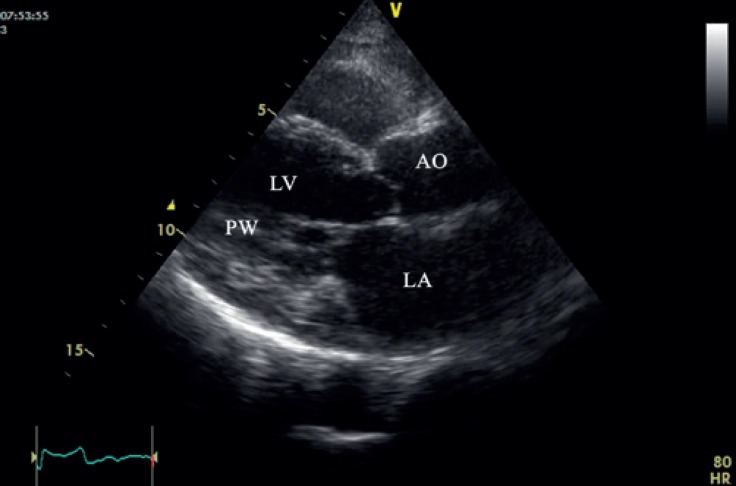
Transthoracic 2-dimensional echocardiography in the parasternal long-axis view reveals a severely thickened pericardium (arrow).
